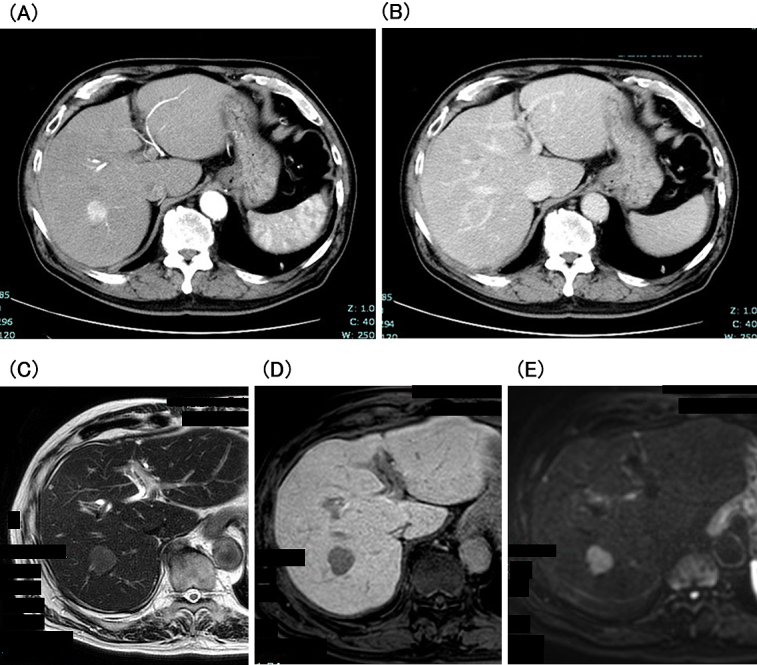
Contrast-enhanced CT shows that the tumor was hyperdense in the arterial phase (A) and hypodense in the delayed phase (B) images. EOB-MRI shows that the tumor was hyperintense on T2WI (C) and DWI (D) and clearly hypointense in the hepatocyte phase (E). EOB-MRI, gadoxetic acid-enhanced magnetic resonance imaging; T2WI, T2-weighted image; DWI, diffusion-weighted imaging.
